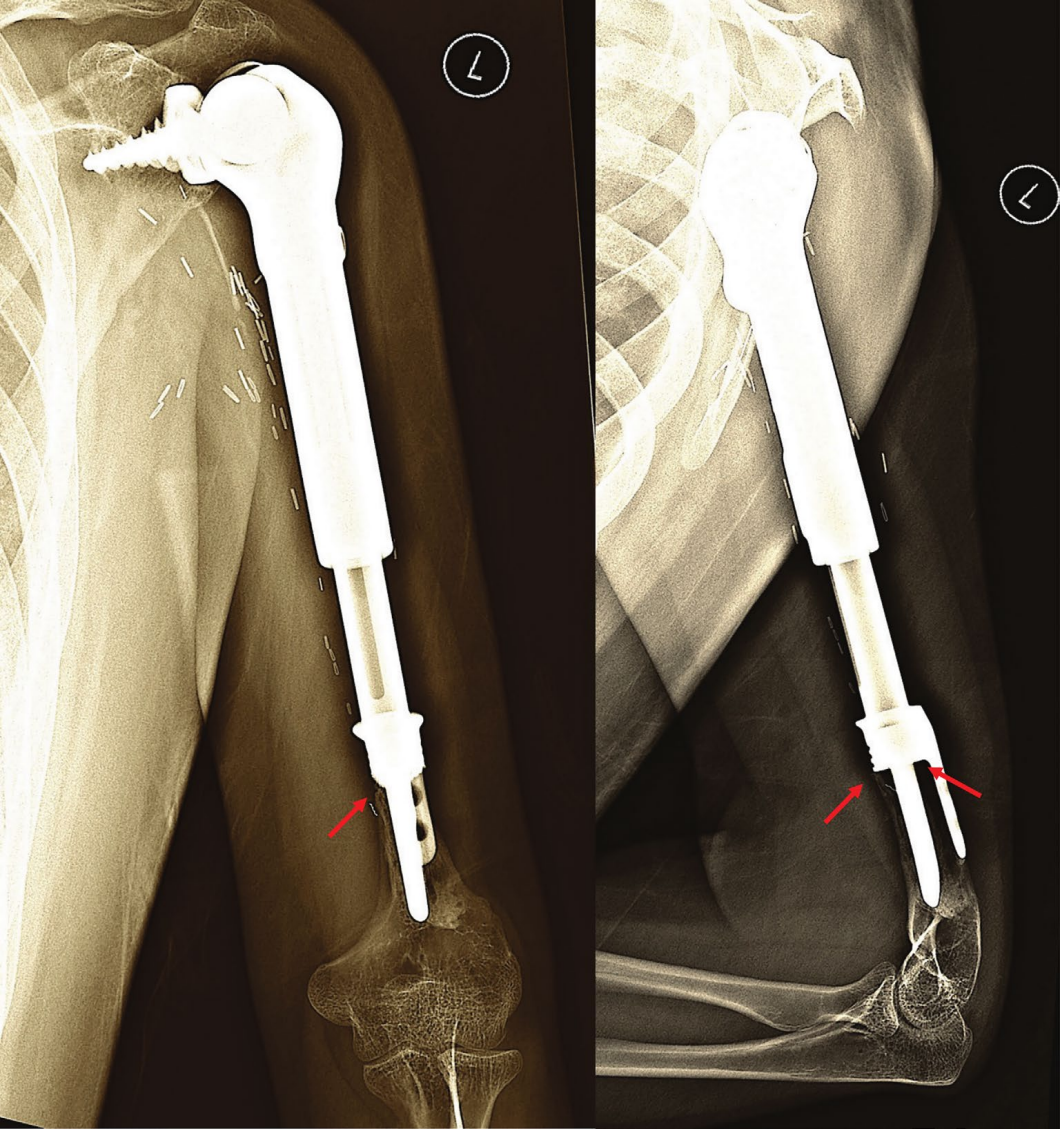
AP and lateral views of the most recent plain radiographs of patient in Figure 7 with the growing (extendable) endoprosthesis showing contact between the cortical bone and stem collar (red arrows) but no extracortical bone bridge ingrowth. However, no radiolucent lines are present to suggest loosening.
